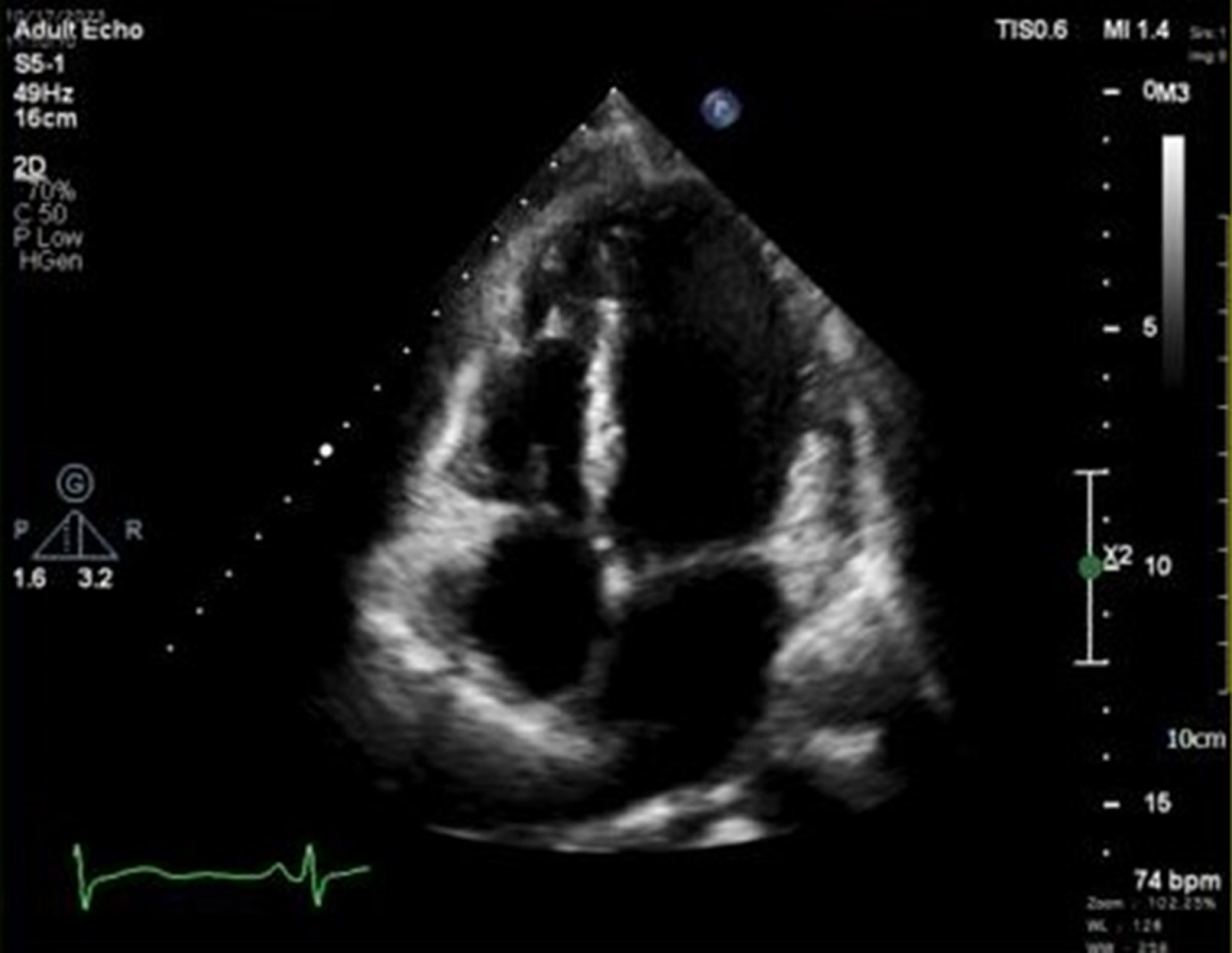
TTE of a patient with papillary fibroelastoma of RA, underwent mass resection and TV repair. Complete surgical excision resulted in the absence of residual mass or thrombus in the RA, with a normal size, and mild to moderate systolic dysfunction. Mild AI, MR, and TR are seen. Mildly thickened and dome‐shaped PV is reported. Also, mild pericardial effusion and hematoma without hemodynamic effect, besides the Left side pleural effusion are seen. AI, aortic insufficiency; MR, mitral regurgitation; RA, right atrium; TR, tricuspid regurgitation; TTE, transthoracic echocardiography; TV, tricuspid valve.
